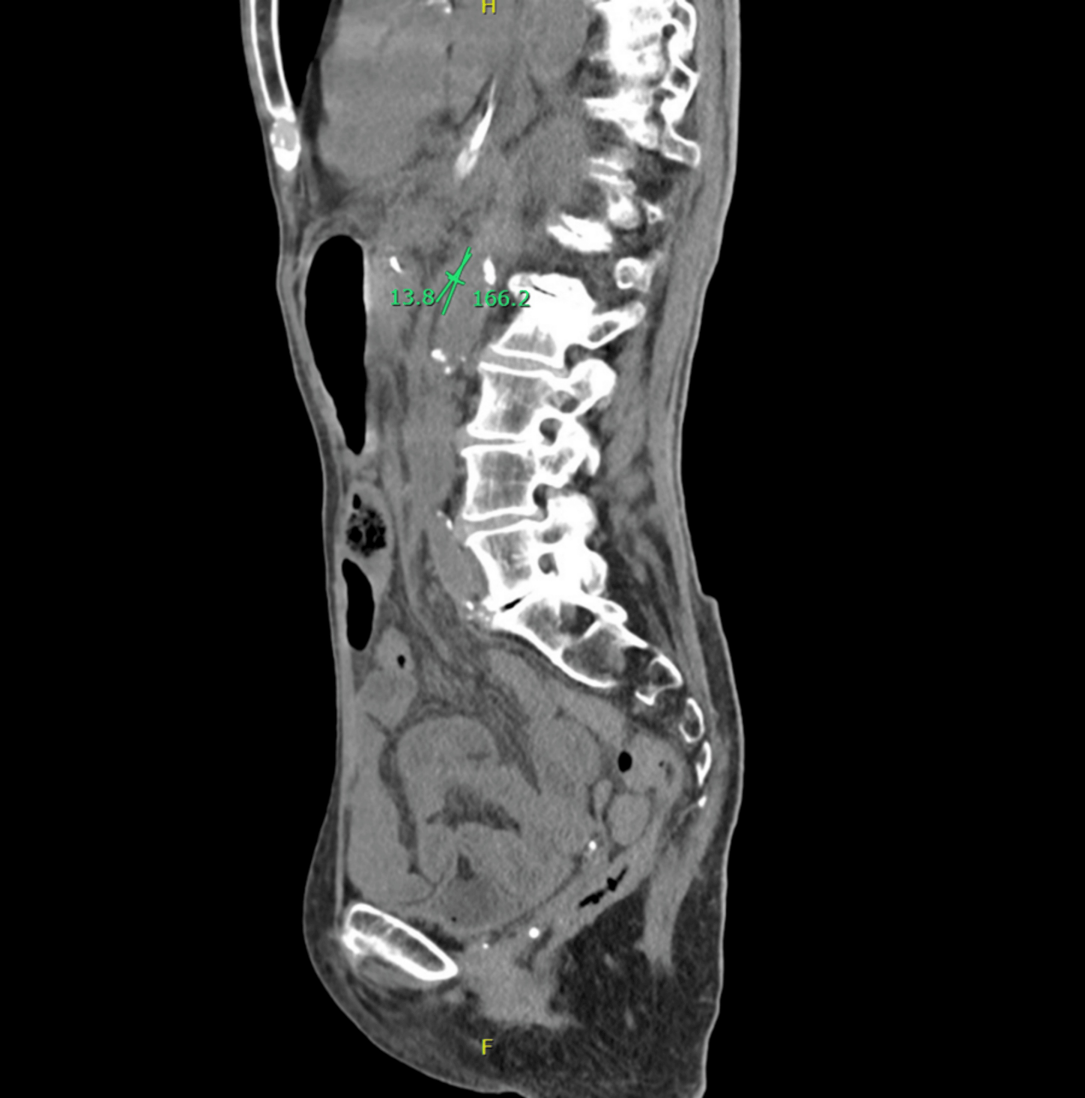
Sagittal view of abdominal CT showing a decrease in the aortomesenteric angle of 14°. CT: computed tomography
